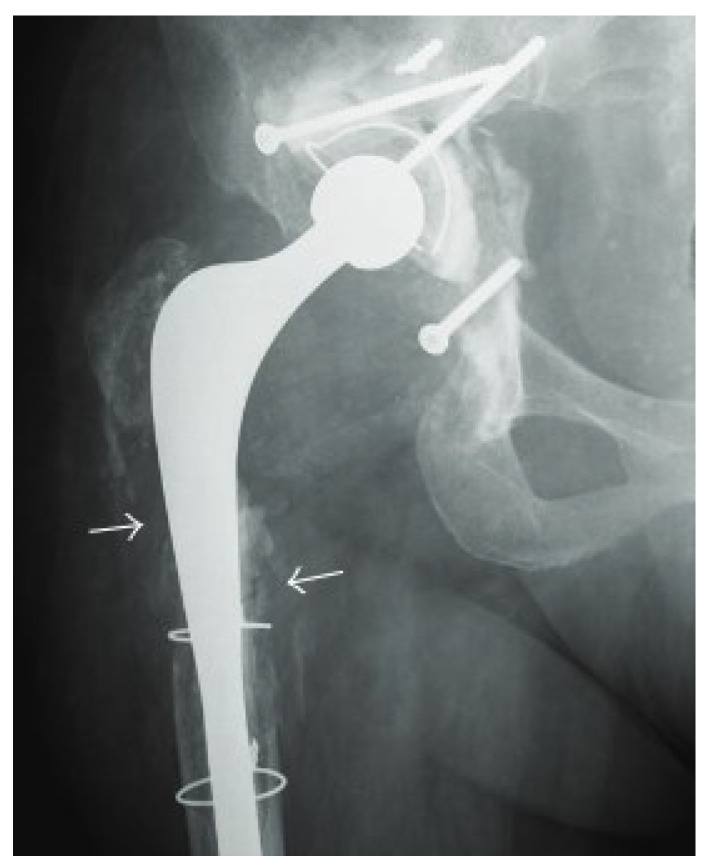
X-ray of PJI after hip arthroplasty; X-ray shows lytic lesions surrounding the femoral component (arrows) [50].
